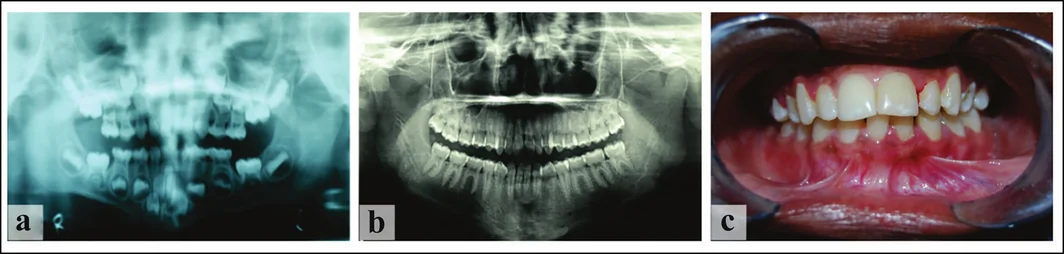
Long‐term follow‐up demonstrating favorable mandibular growth and dental development. Left: Panoramic radiograph at 4 years of age. Center: Panoramic radiograph at 18 years of age. Right: Intraoral appearance at 18 years showing stable occlusion and satisfactory healing of the lower anterior vestibular soft tissues.
